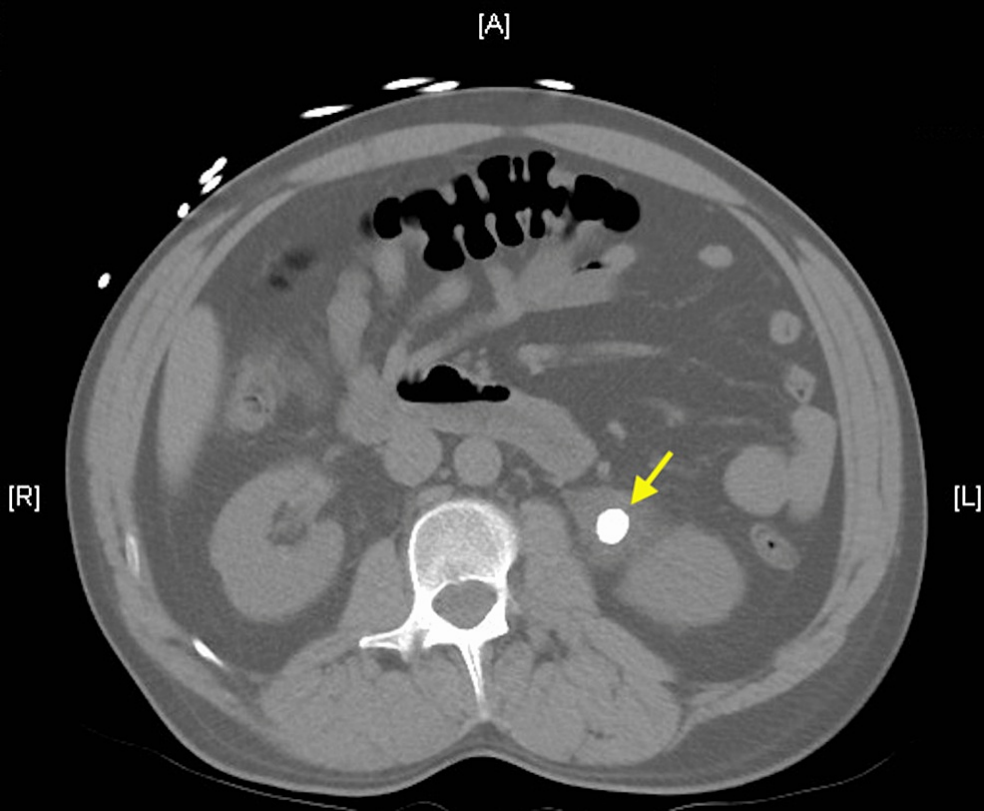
Computed tomography scan visualizing a 12 mm kidney stone in the ureteropelvic junction.
